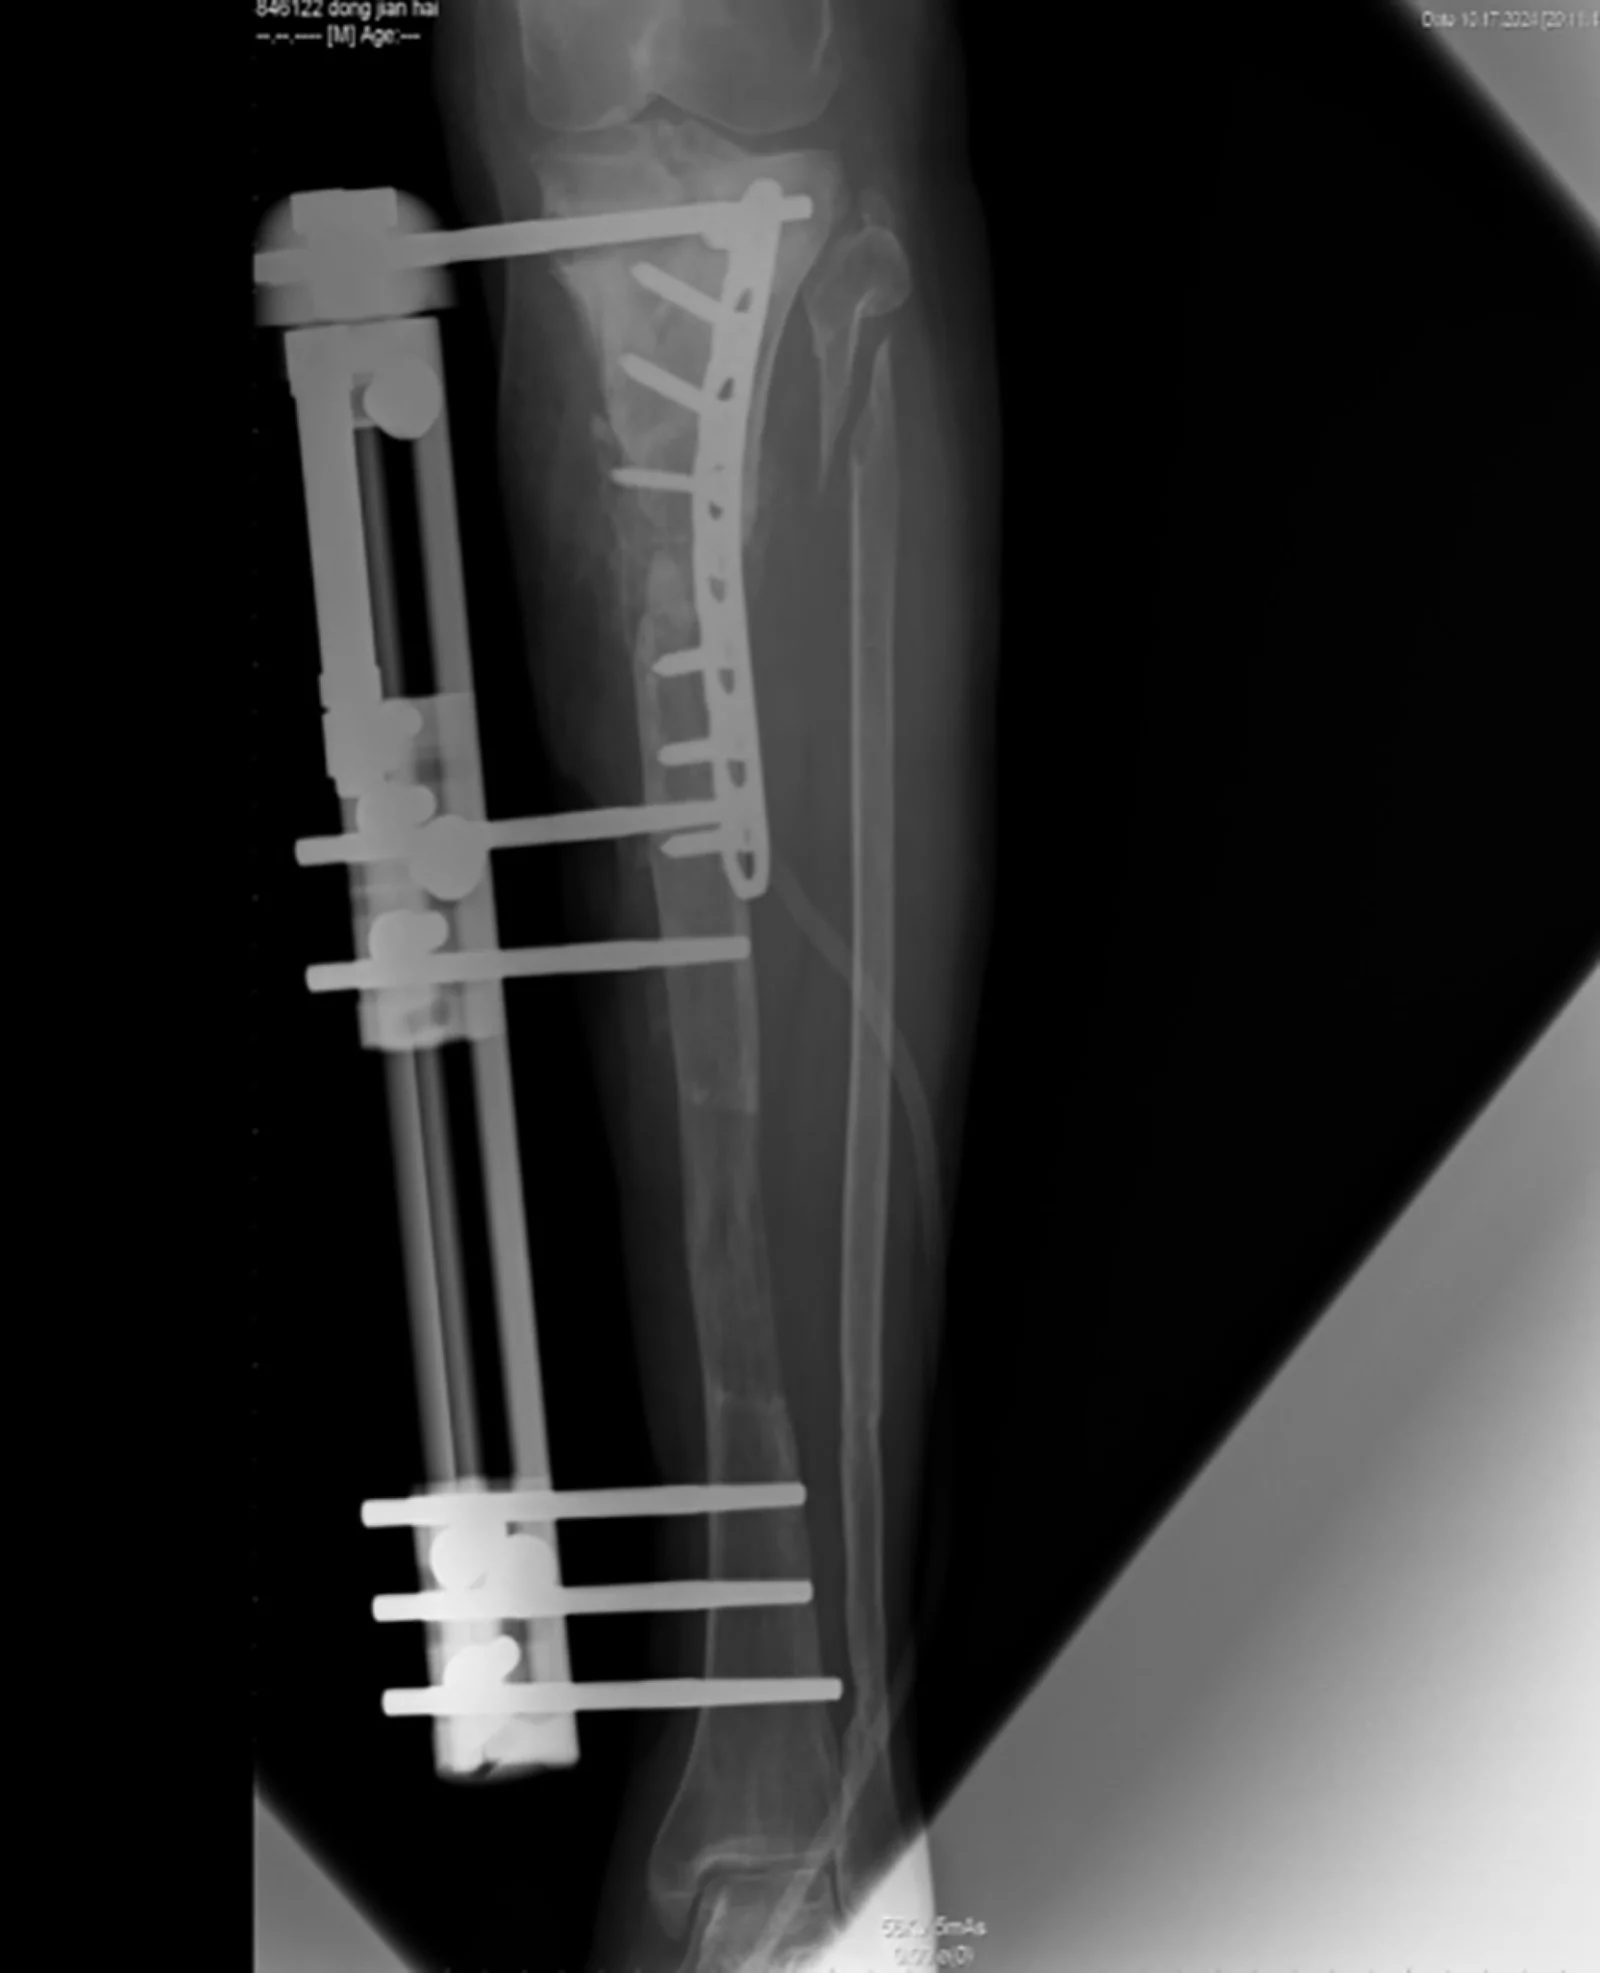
Immediate postoperative radiograph after the final surgery (October 17, 2024). The image shows the status after docking-site revision, autologous iliac bone grafting, and internal fixation. The bone transport segment has successfully reached the distal end, and the regenerate bone in the distraction gap shows satisfactory mineralization.
